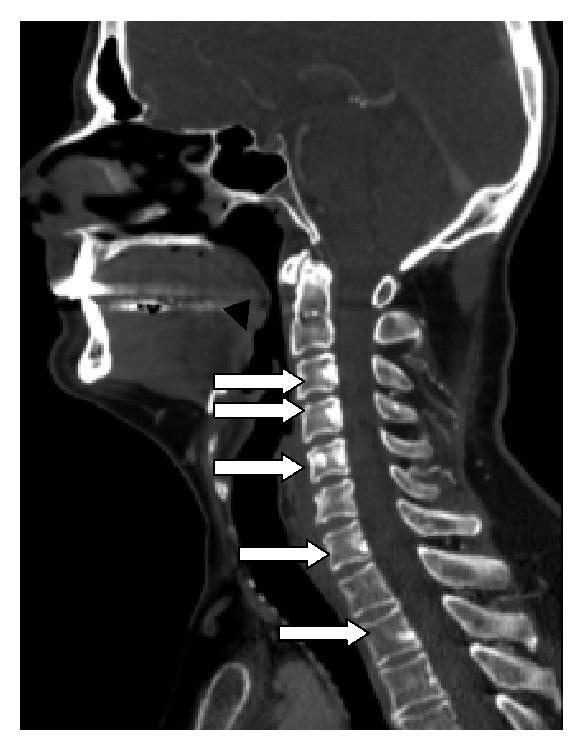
(Neck contrast enhanced CT)*: Sagittal bone window CT demonstrating several osteoblastic lesions at C3, C4, C5, C7, and T2 (white arrows).
